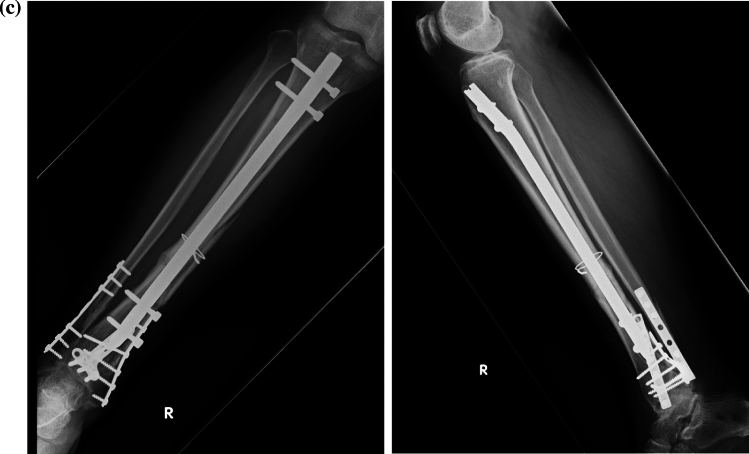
Representative case of a 39-year-old woman involved in a motorbike accident. a Radiographs and CT of a right tibia AO/OTA 42B2 + 43C3.3 fracture. b Post-operative radiographs after the fracture was operated with a nail-plate construct. c Radiograph 6 months after the operation showing bone union. CT: computed tomography, AO/OTA: Arbeitsgemeinschaft für Osteosynthesefragen/Orthopedic Trauma Association
